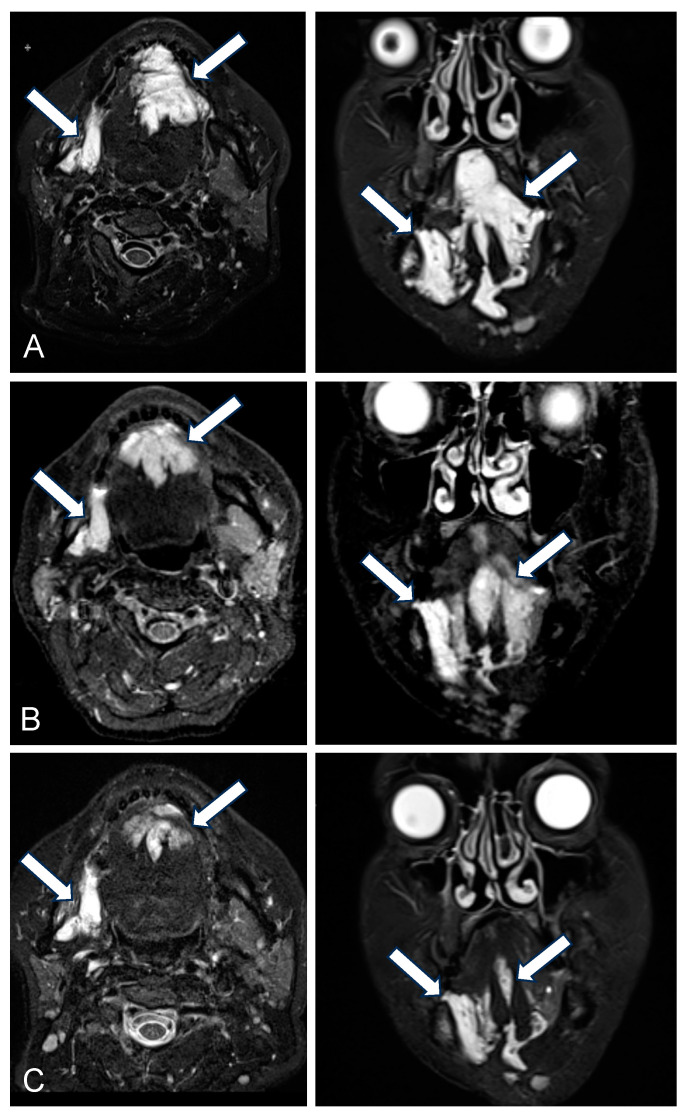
A 49-year-old female patient with an extensive venous malformation (VM, indicated by white arrows) of the oral cavity and tongue undergoing 3 sequential bleomycin electrosclerotherapy (BEST) sessions with intralesional drug administration. (A) T2-weighted (T2w) axial and coronal magnetic resonance (MR) images obtained prior to BEST treatment, with indications including swelling, pain, dysphagia, and impaired speech. (B) T2w axial and coronal MR images acquired 3 months following the second BEST session, demonstrating partial regression of the malformation. (C) T2w axial and coronal MR images 6 months after the third BEST session, showing near-complete resolution of the malformation. Following the third session, no further swelling or pain was reported, and lesion regression led to the restoration of unimpaired food intake and speech.
